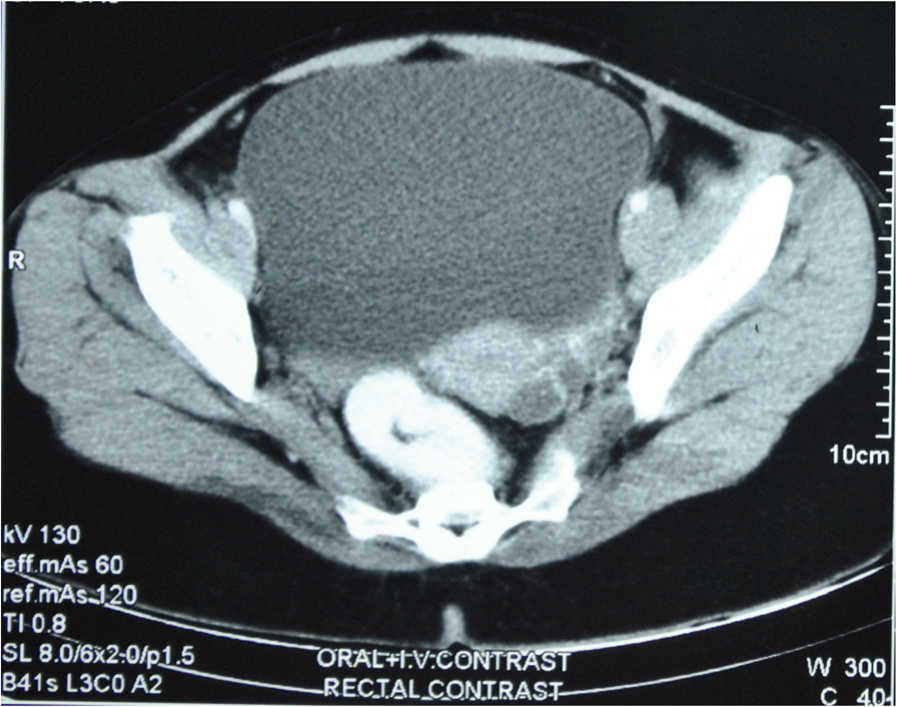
Computed tomography image showing collection in the uterine cavity with lesion in the left adnexa
